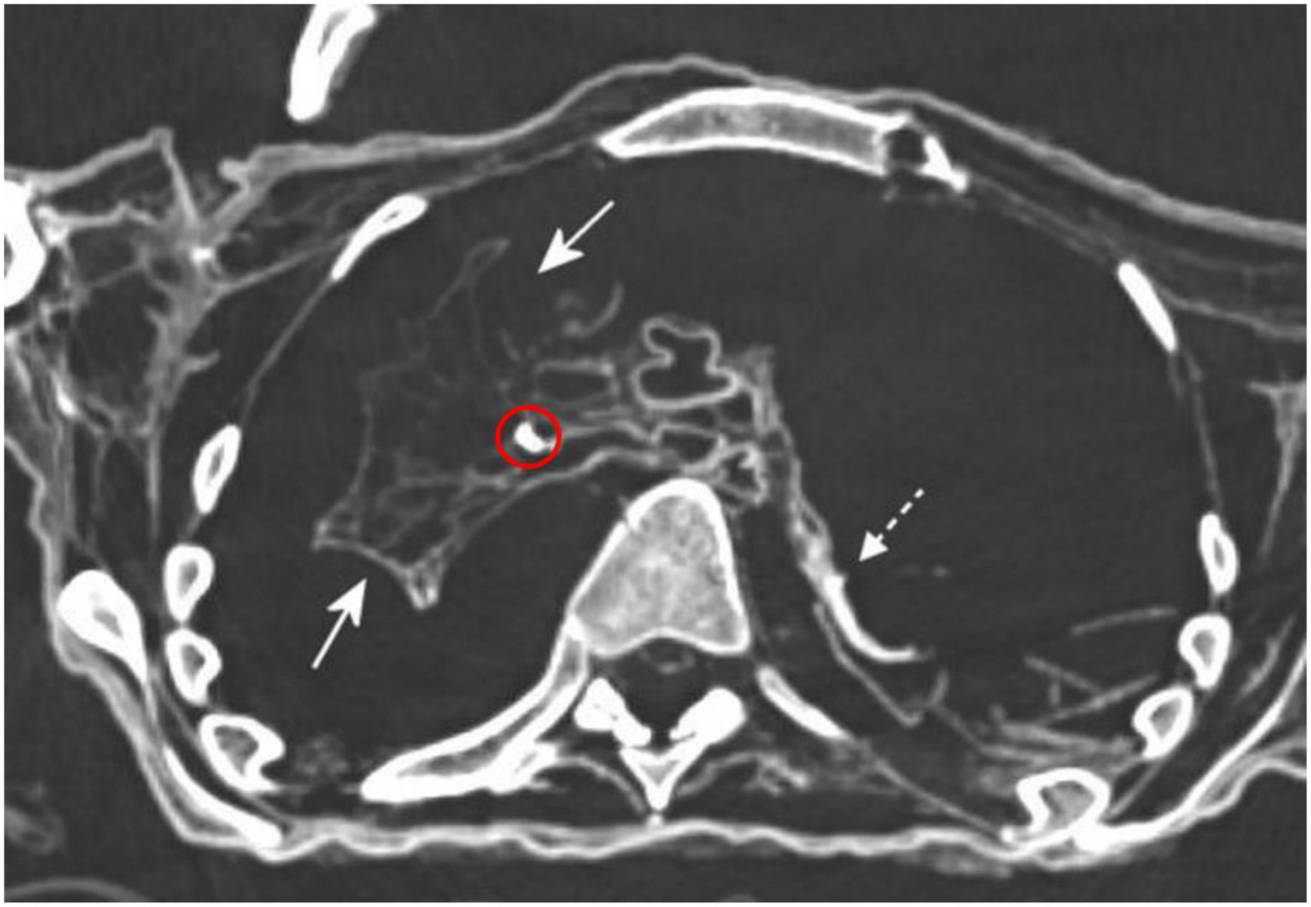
Axial CT reconstruction of the thorax. The left lung was collapsed and flat, condensed at the dorsal chest wall (dotted arrow) in contrast to the right lung, which was only partially collapsed, bridged the space (arrows) and contained several focal calcifications (circle) (images: S. Panzer).
